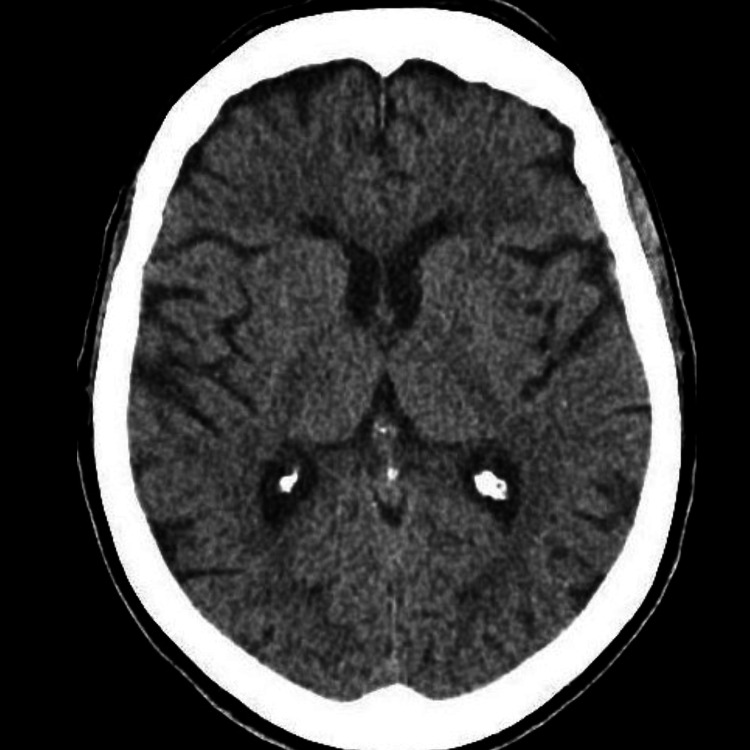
Computed tomography (CT) of the head with normal caudate and thalamus
